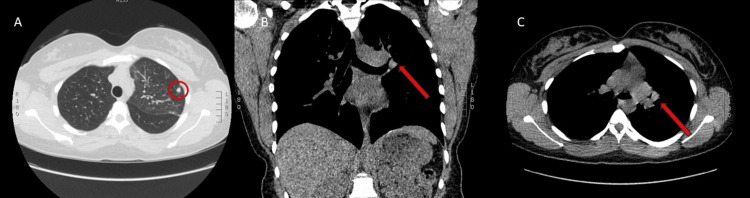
Computed tomography scan A. Axial image demonstrating a 7-millimeter upper lobe nodule. B and C. Coronal and axial images showing perihilar fullness concerning for mediastinal adenopathy and large soft tissue mass in the left paratracheal space with extrinsic compression of the mass on the left main bronchus.
